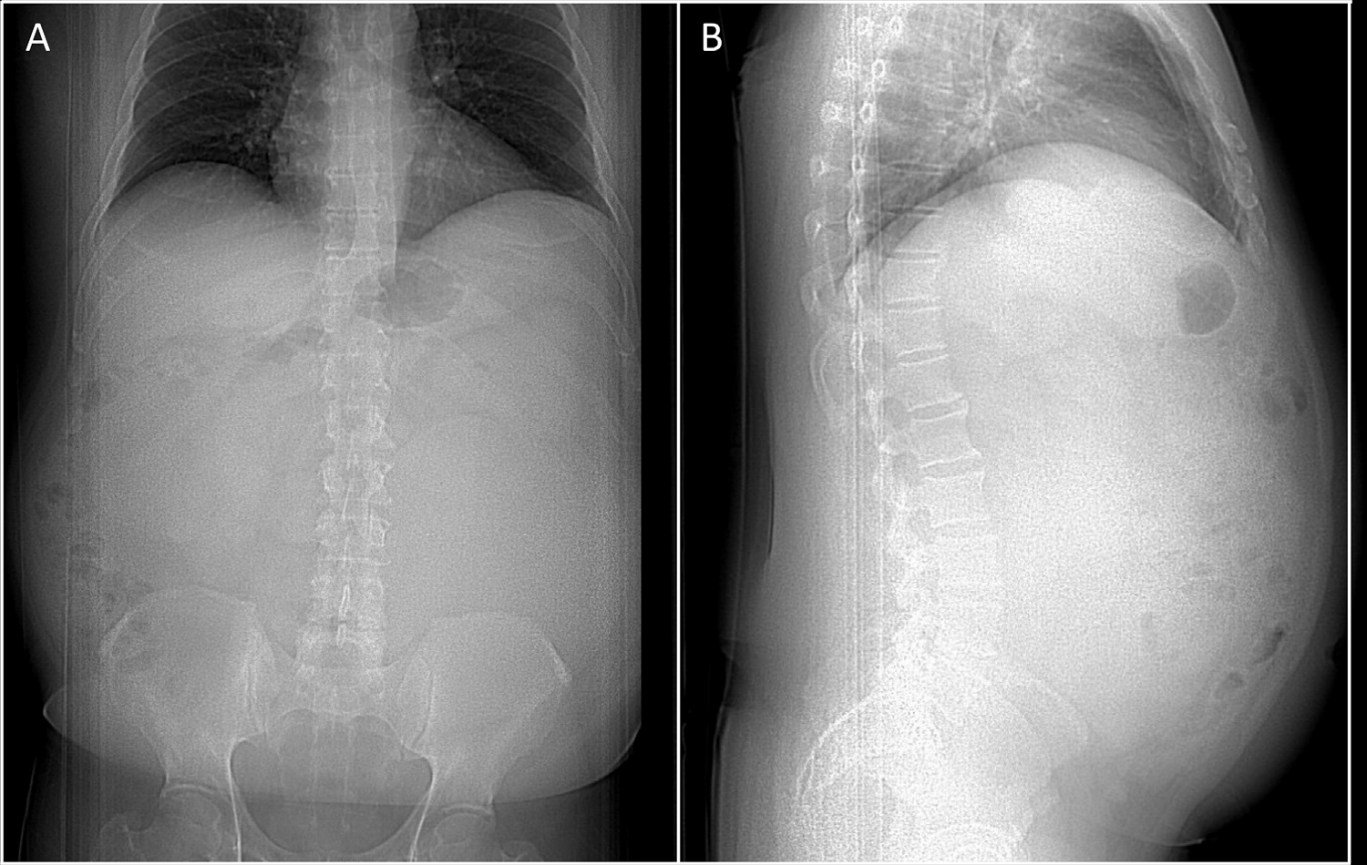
Scout coronal (A) and sagittal (B) CT images of the abdomen and pelvis demonstrate effacement of the iliopsoas muscle shadow and a paucity of bowel gas, suggestive of a potential abdominal mass.
